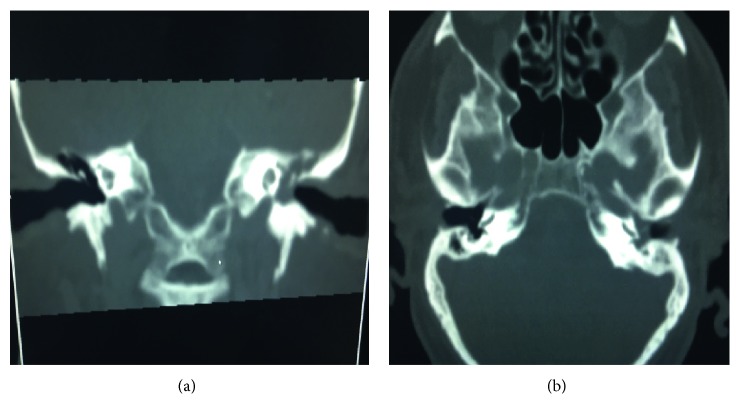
CT scan of temporal bone showing features of tympanomastoiditis and soft tissue shadow involving the middle ear and attic areas. (a) Coronal. (b) Axial.
